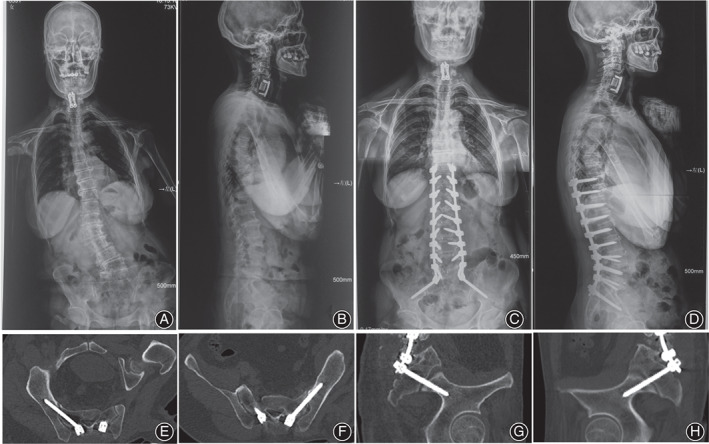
Typical case 2 managed by ball tip technique. A 60‐year‐old female presented trunk shift. She underwent an anterior cervical discectomy and fixation (ACDF) at local hospital 3 years ago. Preoperative X‐rays showed a coronal imbalance. (A, B) Surgeon adopted the ball tip technique for S2AI screw placement. Postoperative X‐rays showed the satisfactory correction and good sagittal and coronal realignments with a sacropelvic fixation (C, D). Postoperative CT scan indicated the perfect positions of S2AI screws bilaterally in the axial and sagittal plates of screws without any cortex breach (E–H).
